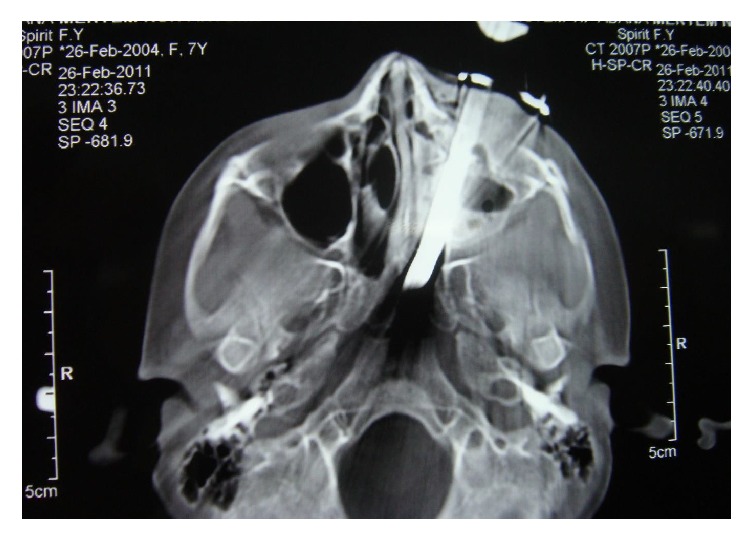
Foreign body visualised on CT at the time of admission.
